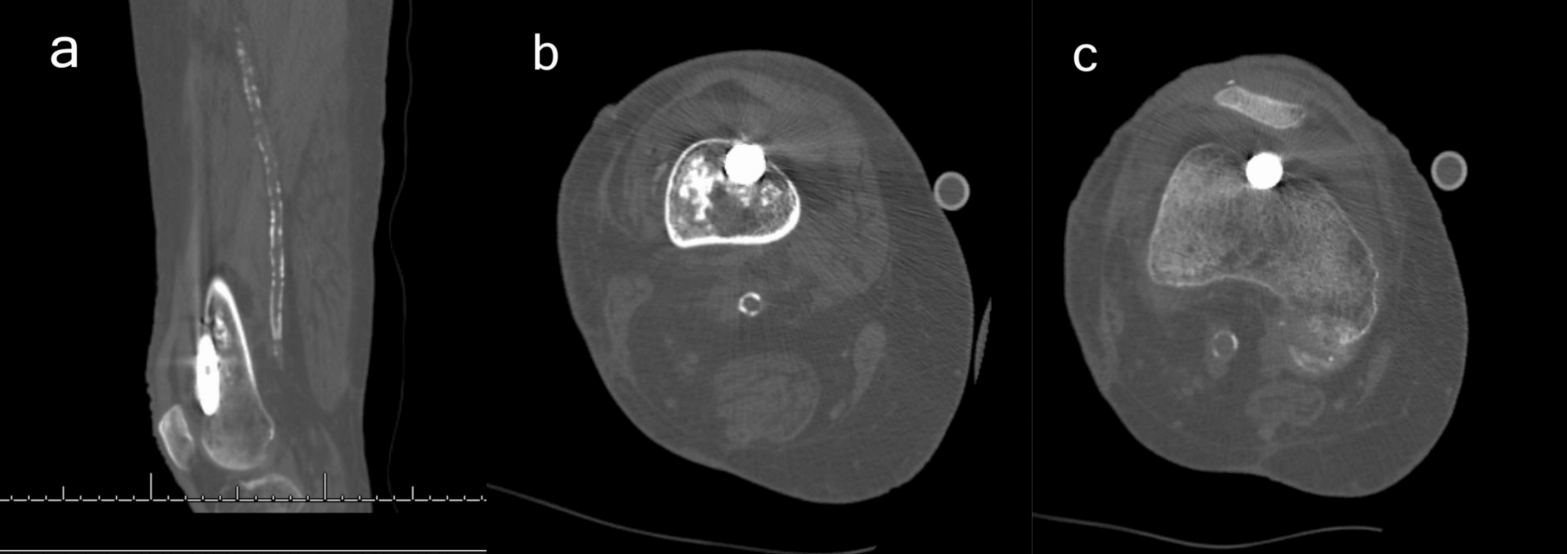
Postoperative CT images demonstrating anterior cortical breach (a) Sagittal cut; (b) coronal cut with sclerotic lesion and anterior cortical breach; (c) coronal cut with anterior cortical breach.
